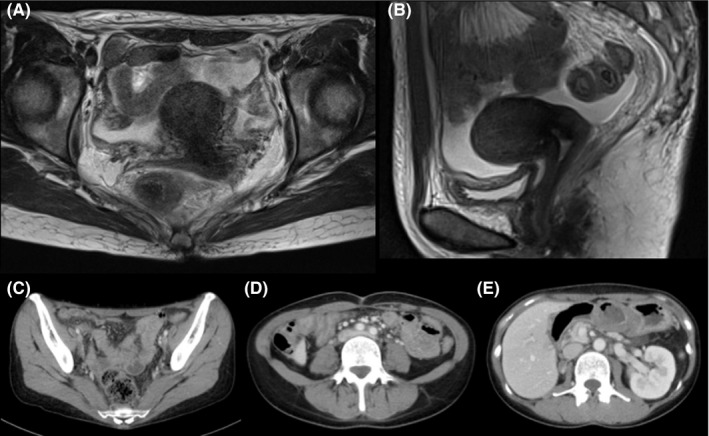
Magnetic resonance imaging (MRI) and computed tomography (CT) after completion of chemoradiotherapy. A, B, MRI of axial and sagittal planes, respectively, showing the uterine cervix. C‐E, CT image showing the external iliac, common iliac, and para‐aortic lymph node regions, respectively
